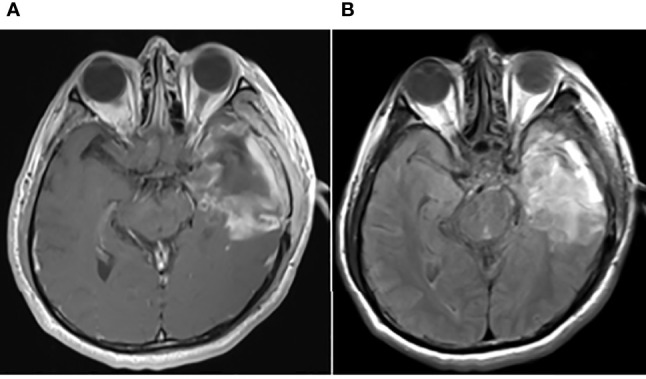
Postoperative brain MRI findings on May 31, 2017. The axial planes of postoperative brain MRI showed that the tumor had been removed. (A) Enhanced T1-weighted MRI. (B) T2-weighted FLAIR MRI.
